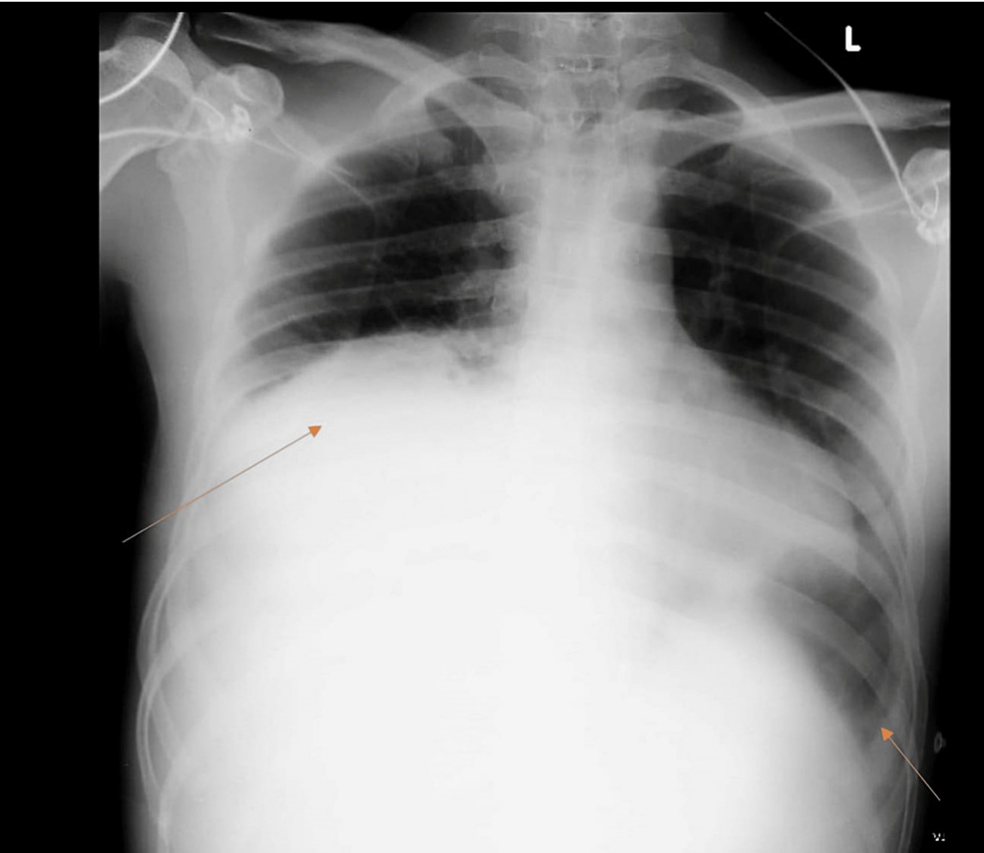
Chest X-ray in anteroposterior (AP) view shows moderate right pleural effusion and mild left pleural effusion (indicated by the arrows)
